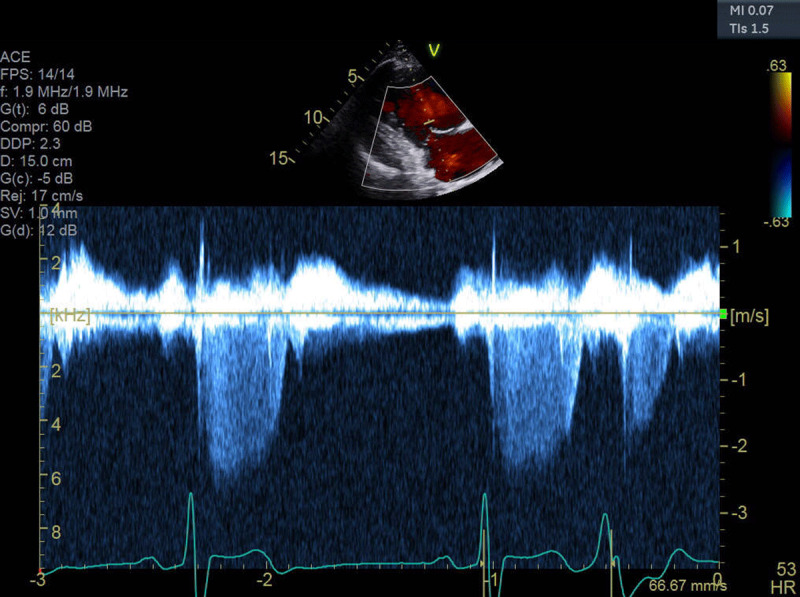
Tricuspid regurgitation velocity by continuous wave Doppler.
